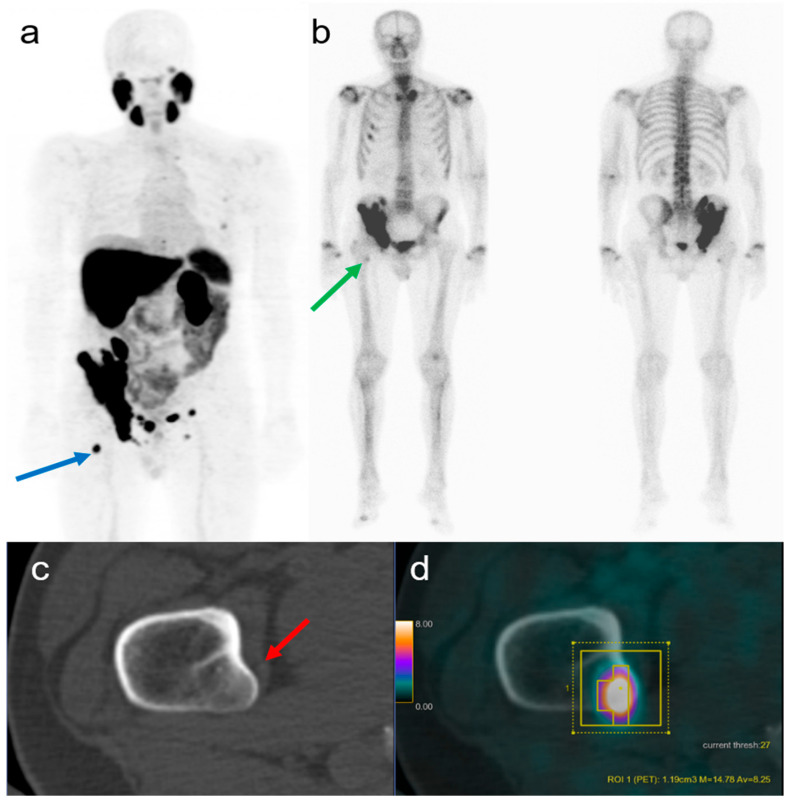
(a) Coronal MIP of a [^68^Ga]Ga-PSMA-11 PET/CT, with a selected lesion in the right proximal femur (blue arrow). (b) Coronal projections of a BS with [^99m^Tc]Tc-DPD acquired 7 weeks before the PSMA PET with increased uptake in the right proximal femur rated as visible by both readers (green arrow). (c) Axial CT scan in the bone window, not showing clear sclerosis or measurable correlate for the lesion (red arrow). (d) Axial [^68^Ga]Ga-PSMA-11 PET/CT showing intense PSMA uptake, with a SUV_max_ of 14.8, SUV_mean_ 8.25 and PSMA_vol_ 1.2 cm^3^; based on the random forest model, the lesion was characterized as visible on BS, despite the absence of evident morphological alterations on the CT component.
